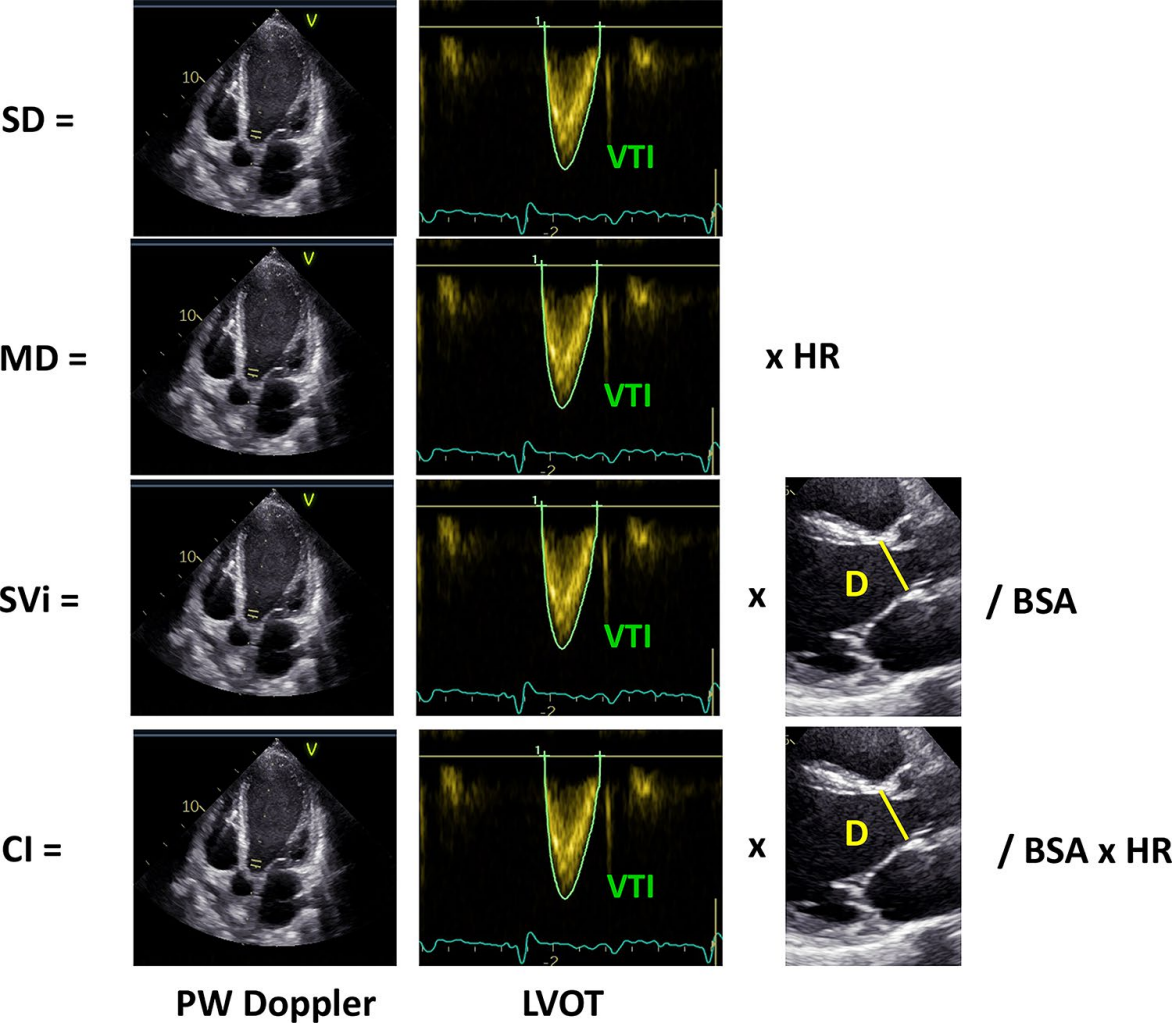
Echocardiographic evaluation of left ventricular blood ejection from the apical 5-chamber view
